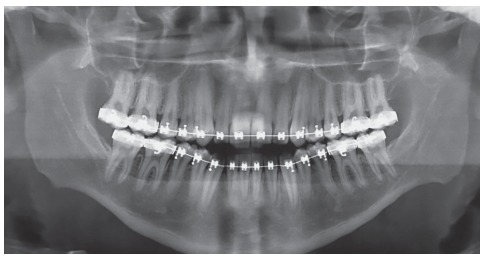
Intermediate: presurgical phase panoramic radiograph.
